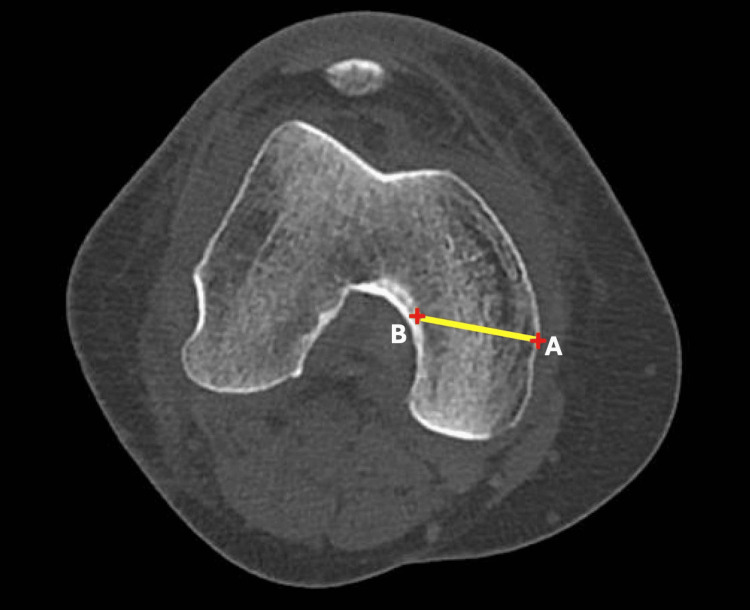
CT image of the distal femur showing mediolateral dimensions of the medial condyle at the distal cut. Line AB: mediolateral dimension of the medial condyle, measured at the level of the distal femoral cut.
